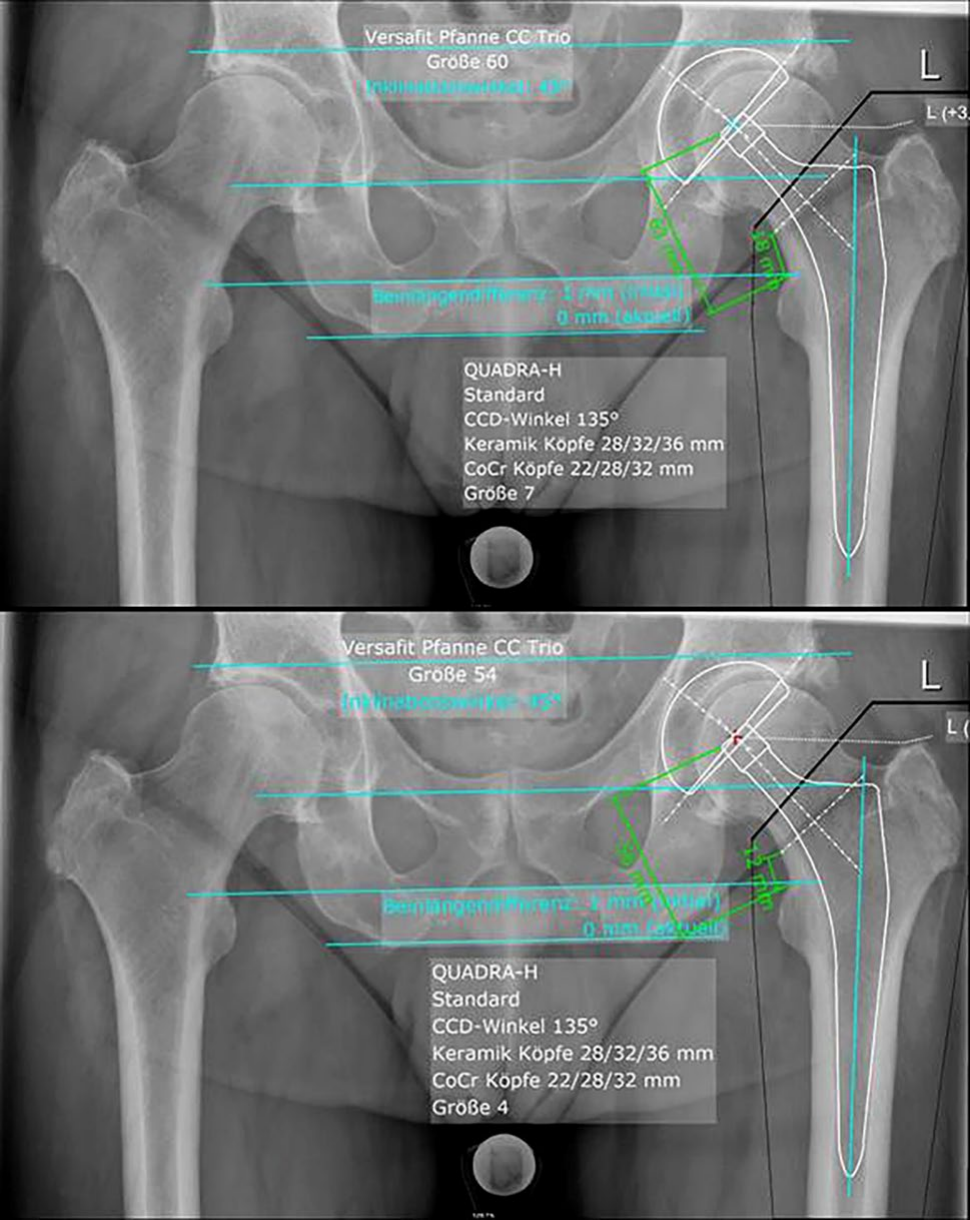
Anteroposterior pelvic radiograph of the same patient scaled with the metal ball 25 mm (top) and the head diameter of the contralateral femoral head obtained from the CT data (bottom) (scaling difference of 11%). Note the distinct difference of the trochanter tip distance of 63 mm (top) to 56 mm (bottom) and the difference in prosthetic component size for both the acetabular cup (60 mm (top) and 54 mm (bottom)) and the stem (size 7 (top) and size 4 (bottom). This scaling difference cannot be detected preoperatively
